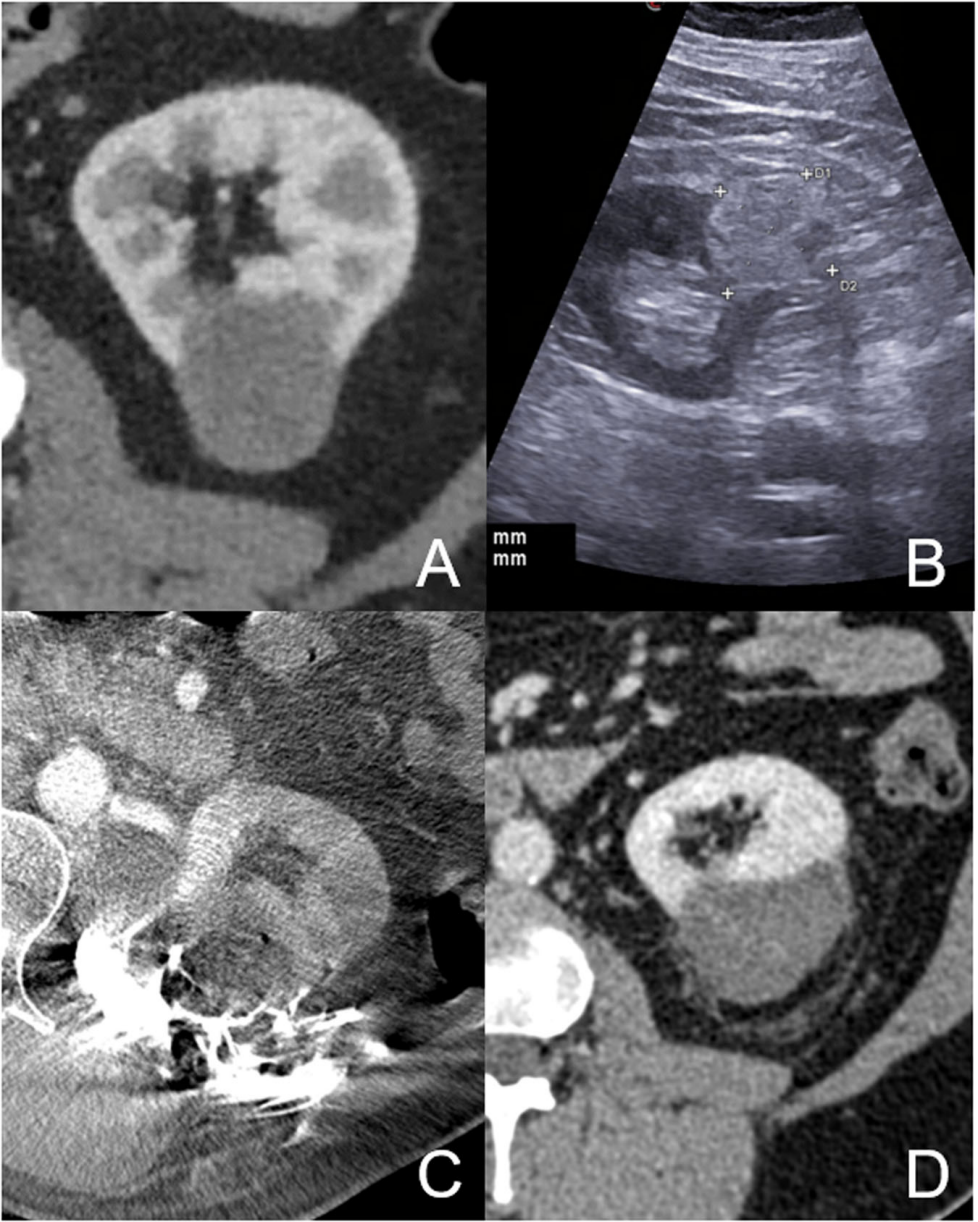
A, B Shows the contrast-enhanced CT in the weeks before the ablation and the native sonogram of a 36-mm papillary RCC at the lower pole of the left kidney. C Shows the intraprocedural cone-beam CT during RFA with ablation for 12 min and hydrodissection. D Shows the CT as a control scan with good local tumor control
